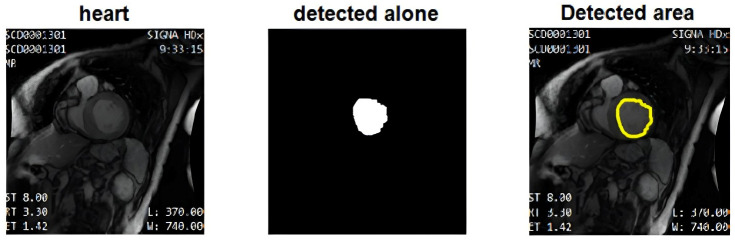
Stepwise refinement. From left to right: (1) Original MRI slice, (2) CNN-derived binary mask of the segmented region, (3) Final refined contour (yellow) overlaid on the original image after post-processing. This figure demonstrates the improvement in boundary smoothness and anatomical coherence achieved through SAE-based refinement and active contour modeling.
